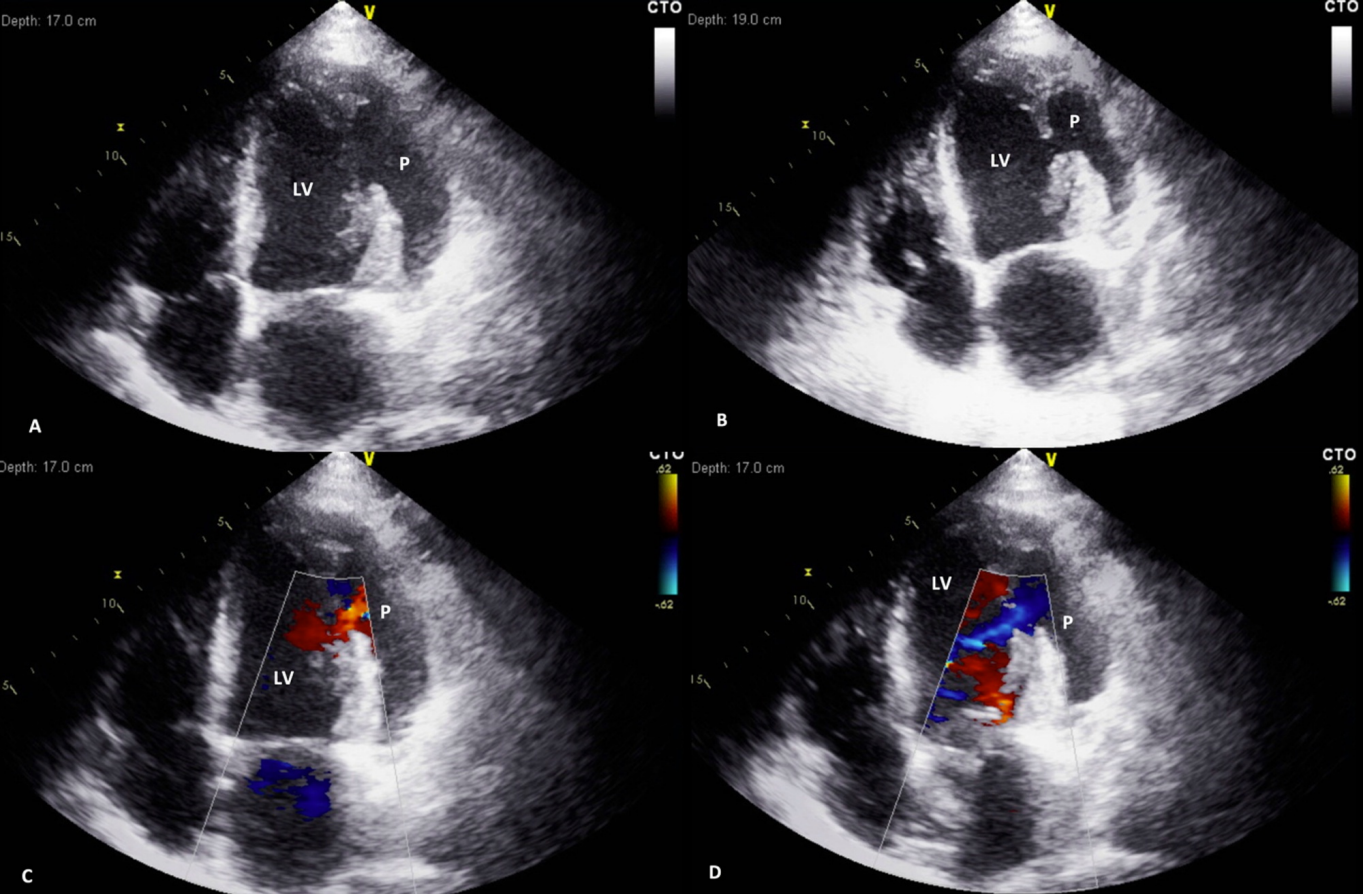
Apical four-chamber views of transthoracic echocardiography demonstrate LV pseudoaneurysm of the lateral wall, severely reduced LV systolic function (<20%), and normal pericardium without effusion (A and B). Color Doppler echocardiography indicates bidirectional flow across a narrow orifice that communicates between the left ventricle and the pseudoaneurysm (C and D). LV = left ventricle; P = pseudoaneurysm
